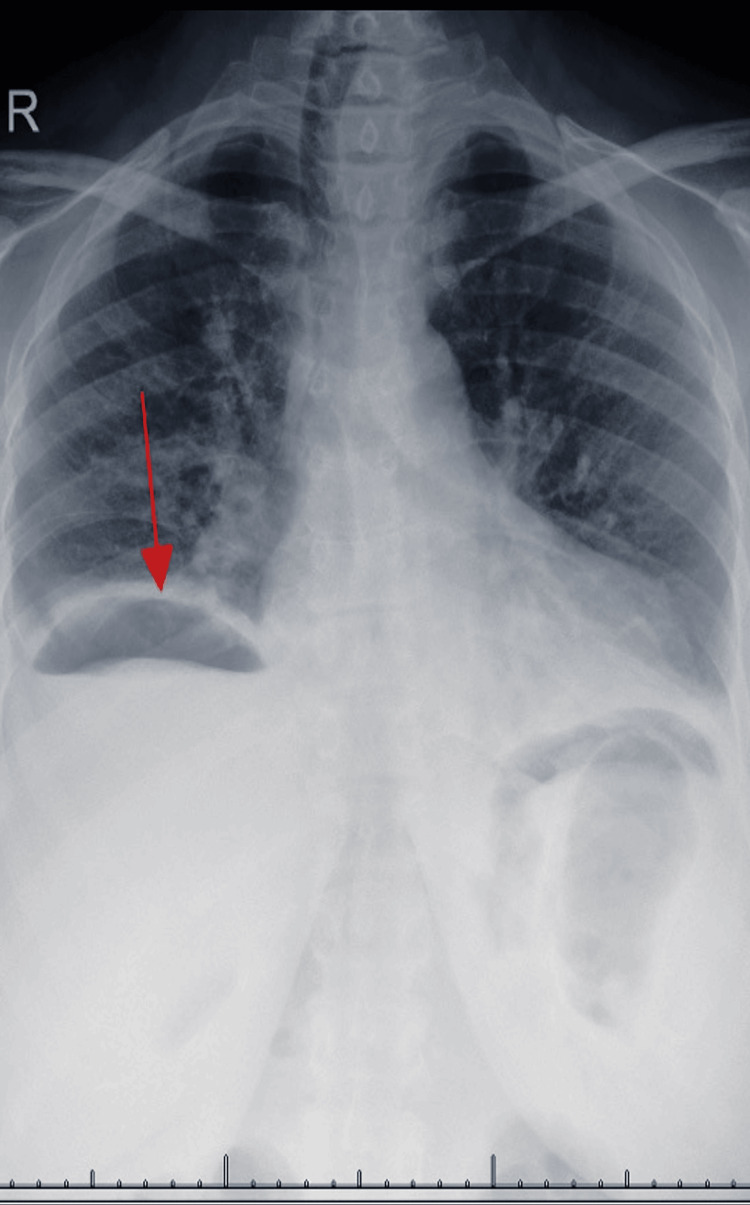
Chest x-ray upright showed evidence of pneumoperitoneum
